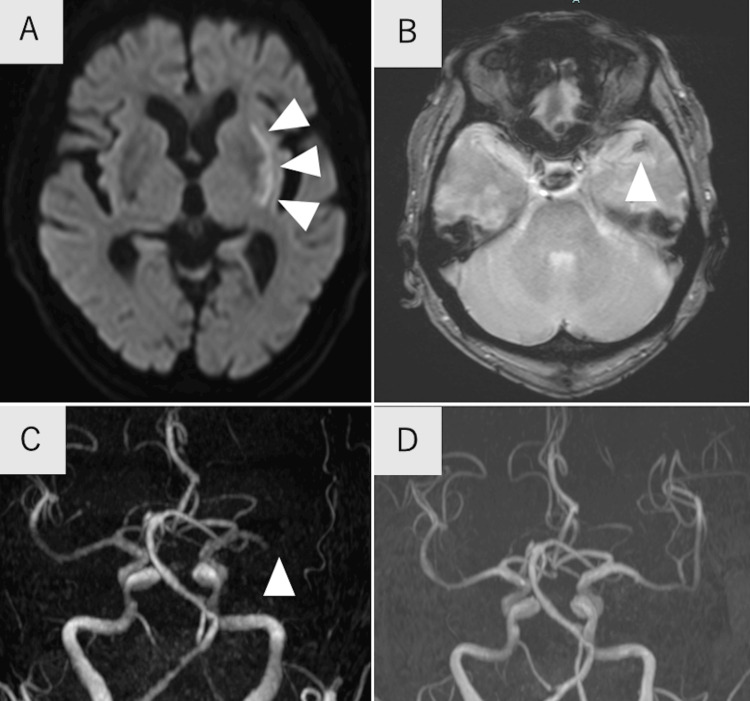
Imaging findings of the recurrent ischemic stroke A: DWI showed a high-intensity lesion in the left putamen (arrowheads). B: T2*-weighted imaging showed a susceptibility vessel sign in the left middle cerebral artery (arrowhead). C: MRA showed left M1 occlusion (arrowhead). D: MRA showed recanalization of the left middle cerebral artery after mechanical thrombectomy. DWI, diffusion-weighted imaging; MRA, magnetic resonance angiography
